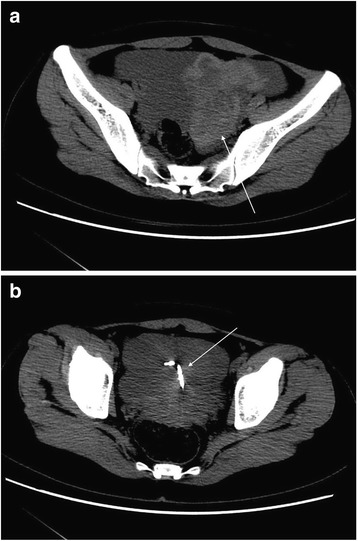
a and b. CT findings of REPWH for the same patient. Patient experienced persistent abdominal pain with no clear cause; HCG test was weakly positive. Left ovarian ectopic pregnancy rupture was confirmed by surgery. The single arrow indicates the mixed-density blood and IUD in the left adnexal area
